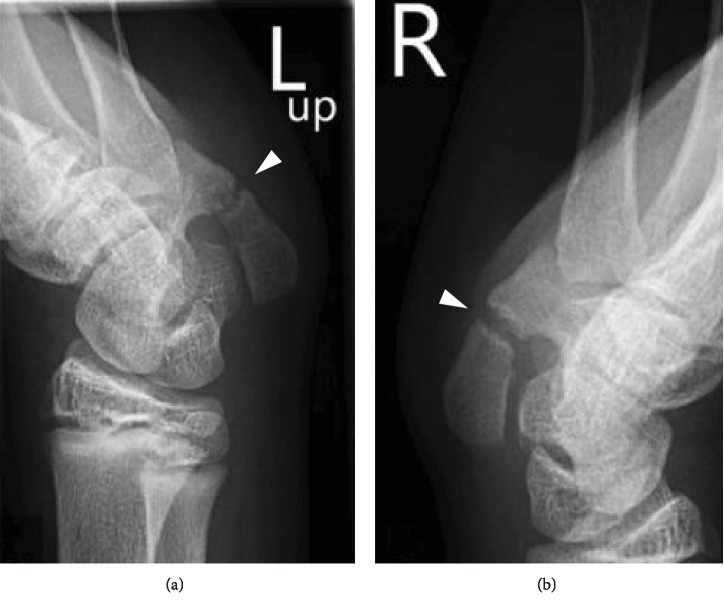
Radiographs of the left wrist (a) and right wrist (b) showing on both sides a coalition between the pisiform and the hook of the hamate (arrowheads).
